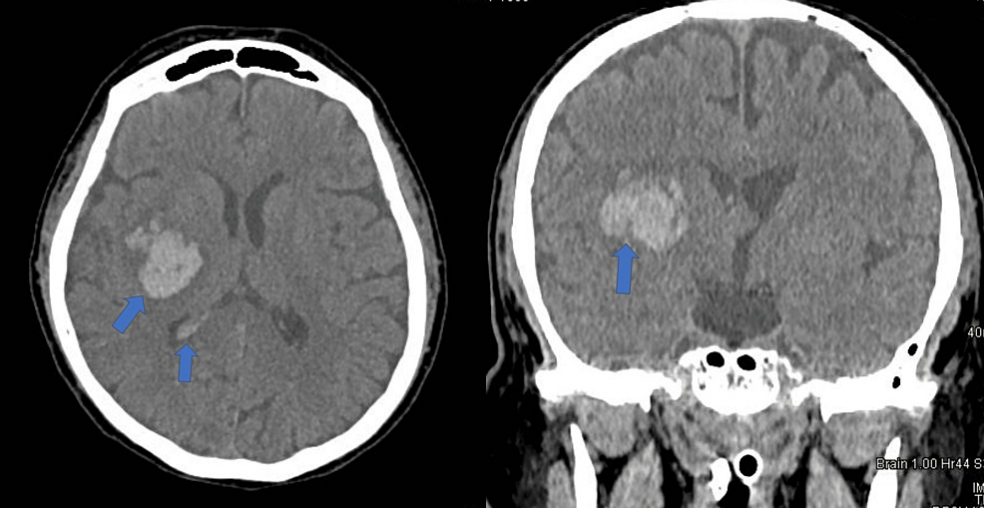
(A) Axial brain CT revealing right basal ganglia intracerebral hemorrhage with intraventricular extension. (B) Coronal CT brain of the same patient revealing right parenchymal hemorrhage with moderate midline shift.
